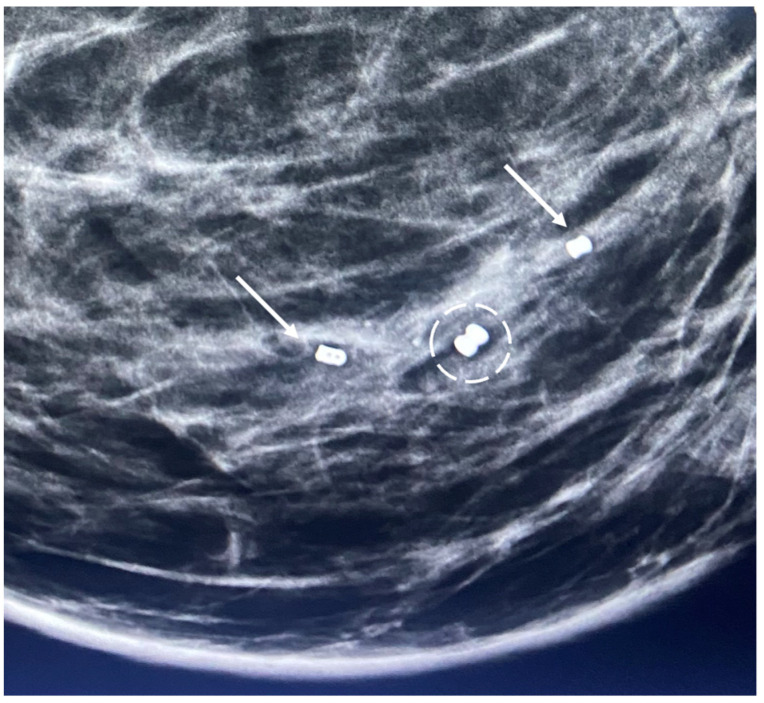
Mammogram showing the metal components (indicated arrows) of two ultrasound-visible biopsy site markers on opposite sides of the original metal biopsy site marker (encircled by hash marks) that was placed at the time of the diagnostic needle biopsy. The original biopsy site marker was not an ultrasound visible.
